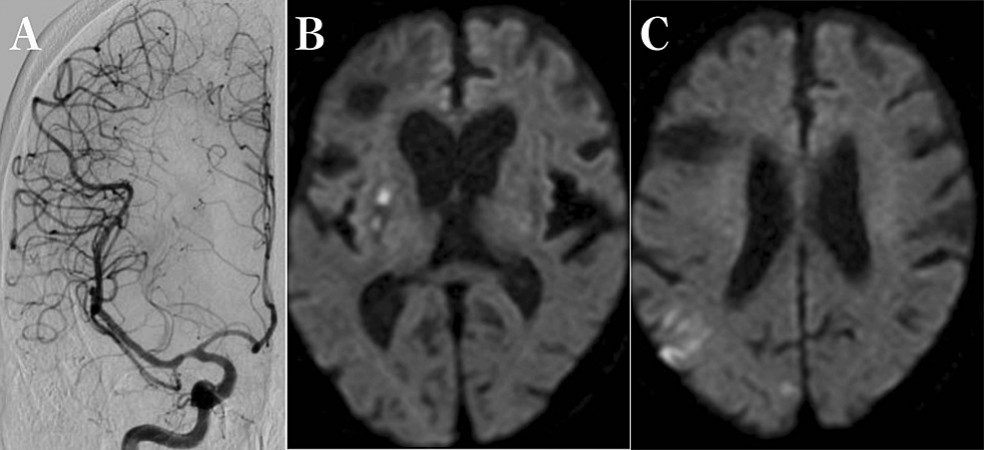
Repeat cerebral angiography and head MRI. A: Right internal carotid angiogram on the fourth day after the procedure. There is no progression of stenosis in the right middle cerebral artery. B, C: Diffusion-weighted images on the eighth day, showing high-signal lesions in the right putamen and part of the parietal lobe.
